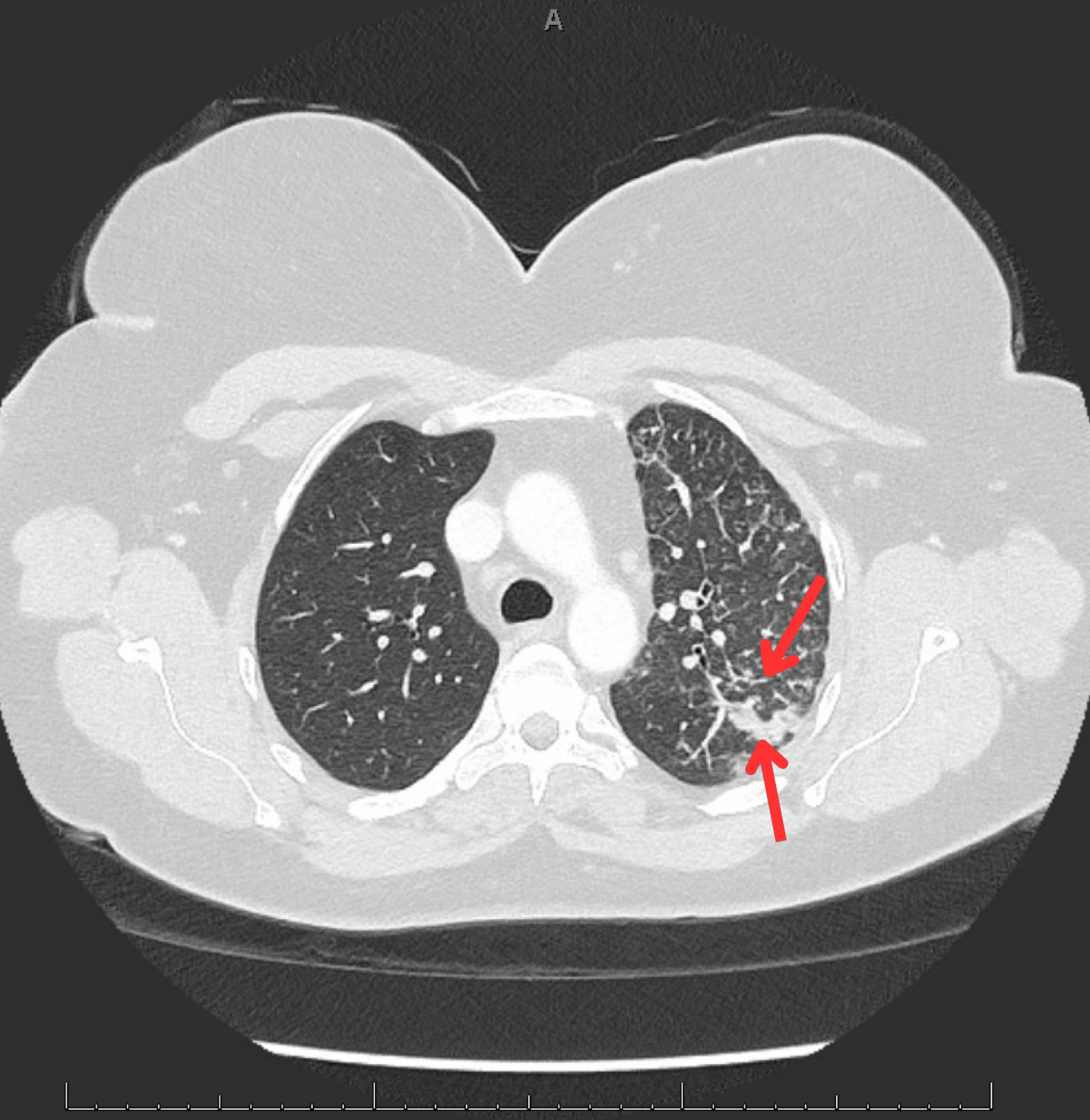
Axial CT image showing tree-in-bud opacities along with consolidative and nodular changes in the upper lobe of the left lung. The red top arrow shows linear opacity with distal airway plugging, and the bottom red arrow shows consolidative changes in the upper lobe of the left lung.
